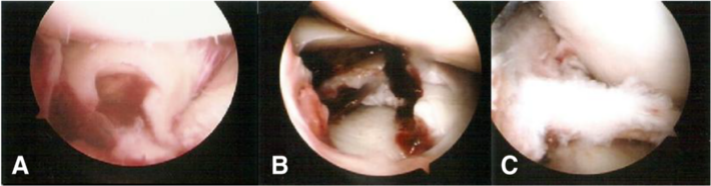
Intraoperative arthroscopic evaluation. a A severely comminuted fracture and a depression of the bicondylar articular surface. b A lateral meniscus tear. c An anterior cruciate ligament (ACL) avulsion fracture. Meyers and McKeever type III. The arthroscopic findings were compatible with the computed tomography (CT) scan findings
